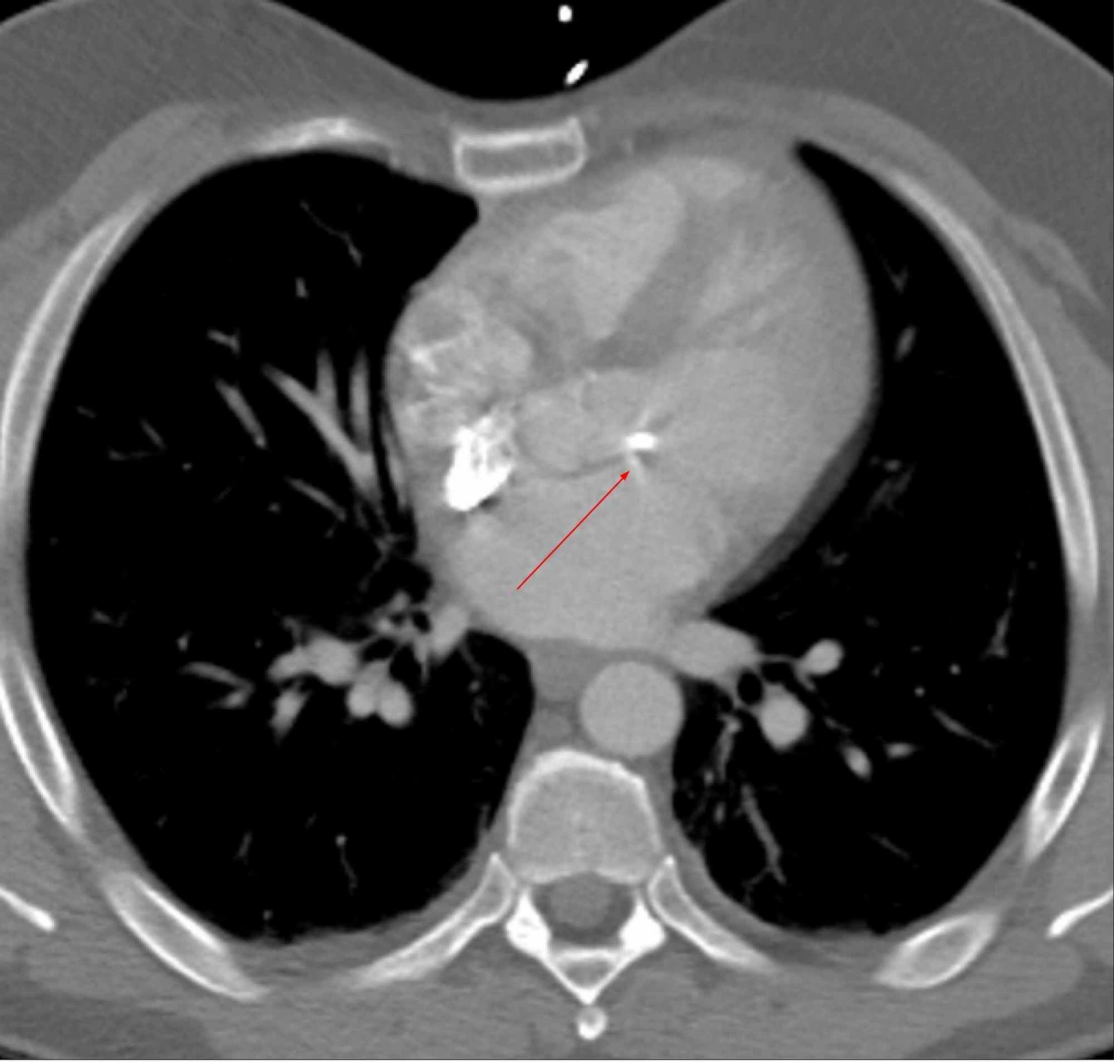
CT of the chest showing calcification of the mitral valve and no pulmonary pathology
